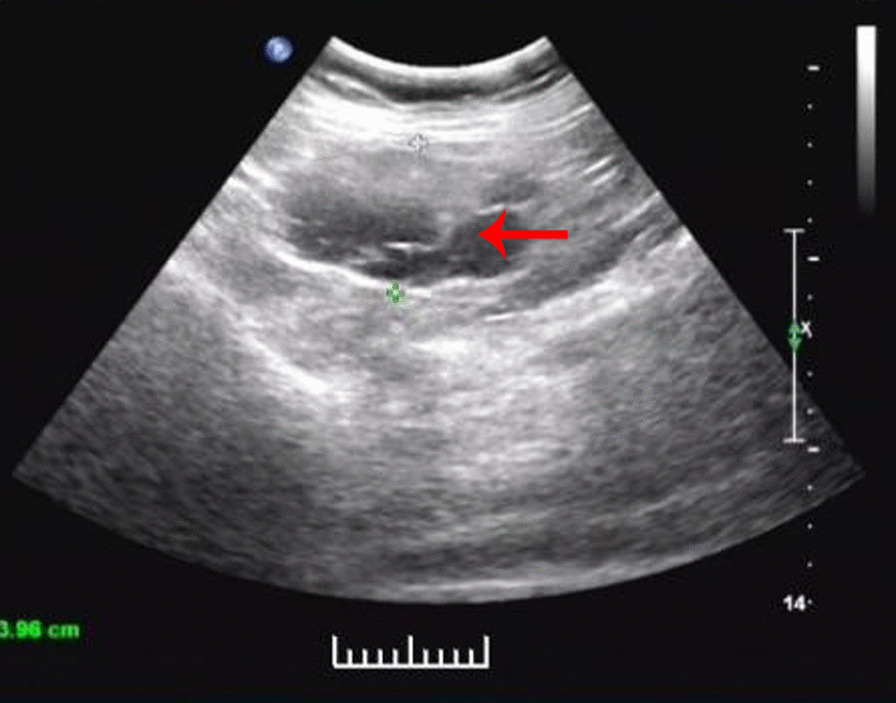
Abdominal ultrasonography showing an abdominal cystic mass of 6.9 cm × 4.0 cm in the left upper and middle abdomen, and colour Doppler imaging showing a spotty colour blood flow signal around the lesion (Case 2)
